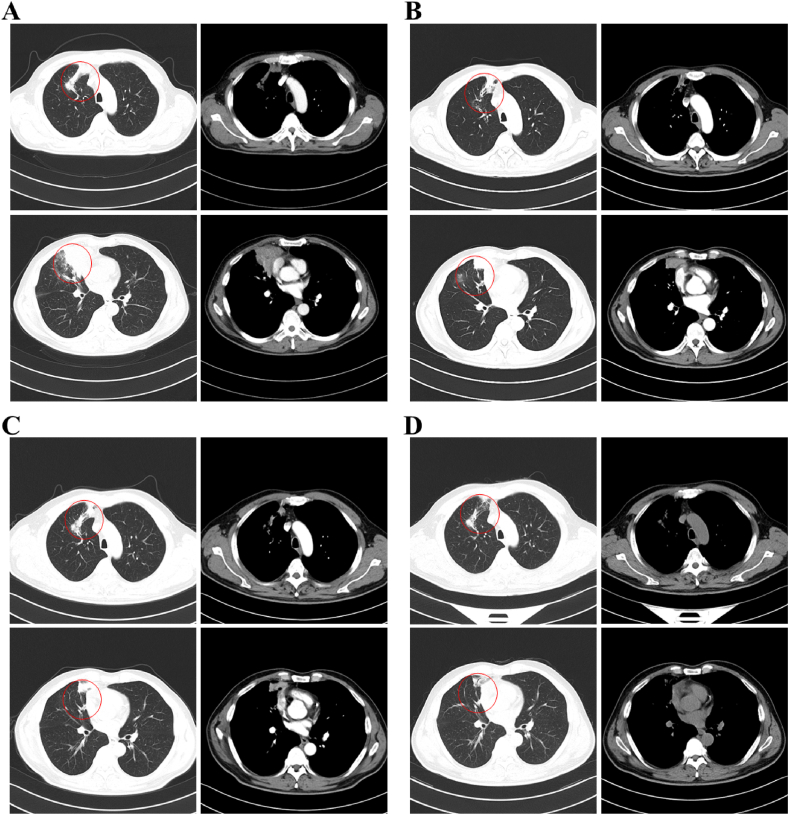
Chest CT scan before and after therapy. (A) Initial chest CT showing multiple lesions in the upper and middle lobes of the right lung, and multiple enlarged lymph nodes in the mediastinum and right hilum (indicated by red circles). (B) Chest CT after 9 days of treatment, showing reduced extent of the upper lobe lesion (indicated by red circles). (C) Chest CT after 18 days of treatment, showing further reduction in lesion extent (indicated by red circles). (D) Chest CT 1 month post-discharge, showing decreased lesion extent and less severe inflammatory changes (indicated by red circles).
